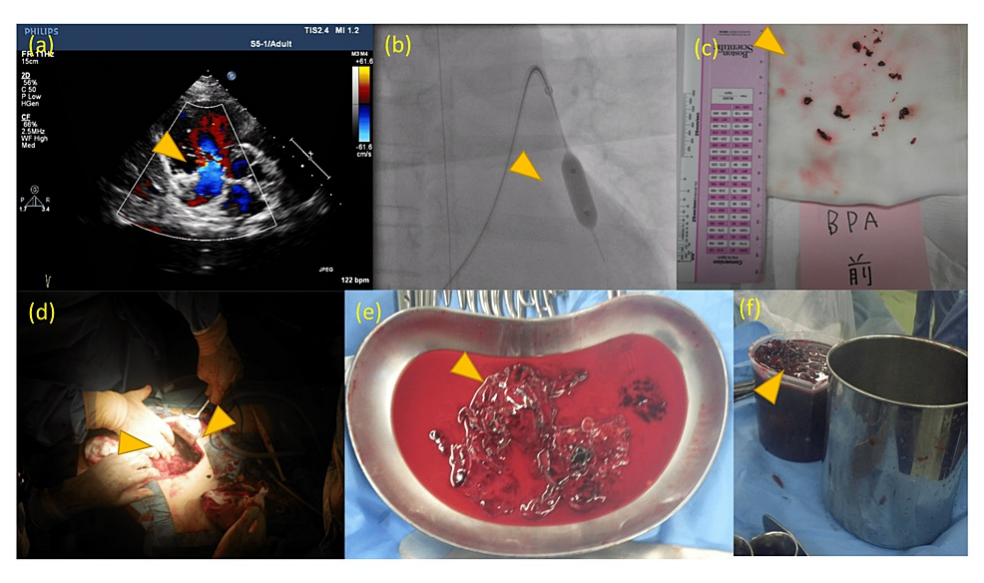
The image of pulmonary embolism treatment. (a) Cardiac ultrasound examination at onset. Right ventricular pressure load was accepted. (b, c) Trans-catheteric removal of the embolism was attempted on day 14. (d, e, f) Intra-abdominal embolism was surgically removed on day 22. Approximately 3920 g of hematoma was removed.
